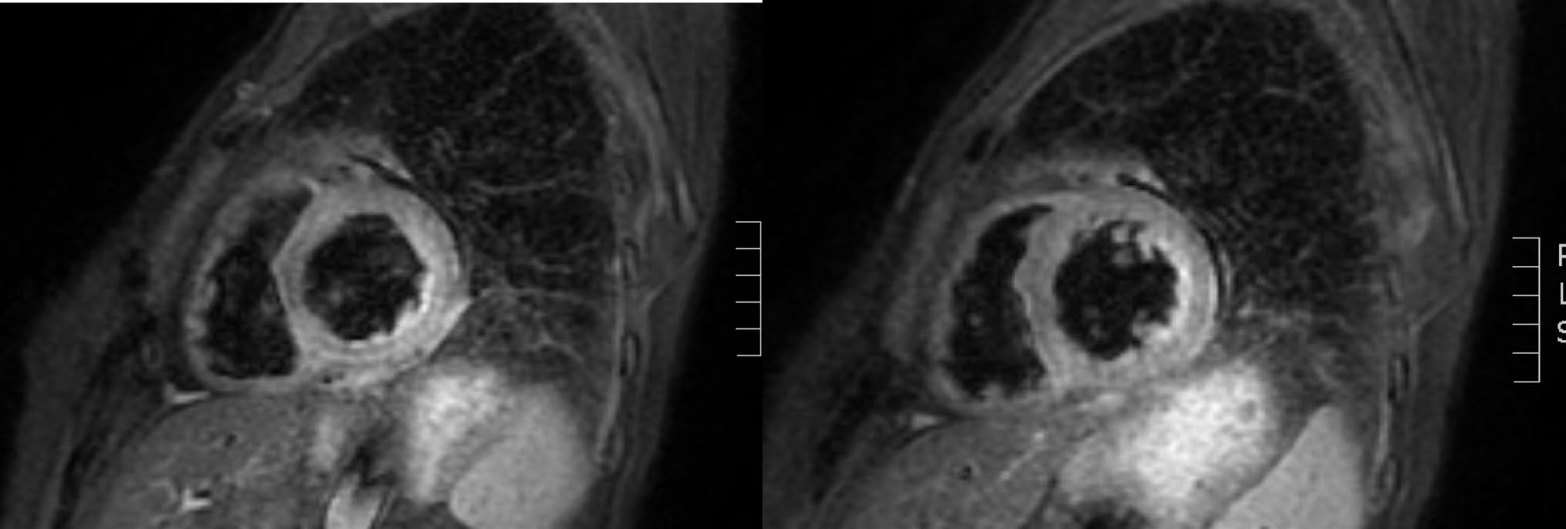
Cardiac MRI findings showing mid-basal left ventricular LGE in a circumferential, subendocardial-to-transmural pattern, more pronounced in the inferior and lateral regions
